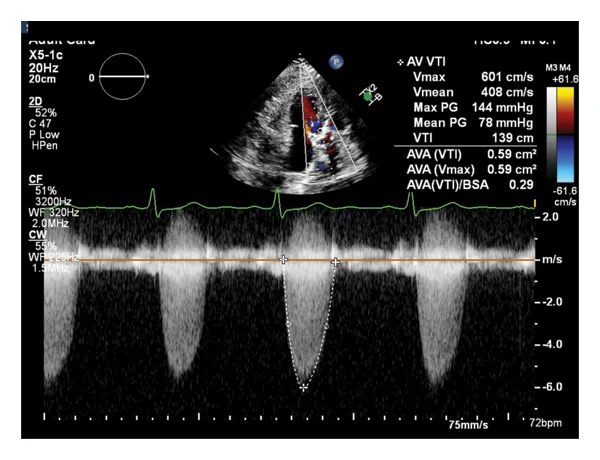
Apical three‐chamber view with continuous‐wave Doppler across the aortic valve, demonstrating the rounded spectral envelope of fixed valvular aortic stenosis and a mean transaortic gradient of 78 mmHg, consistent with severe aortic stenosis.
